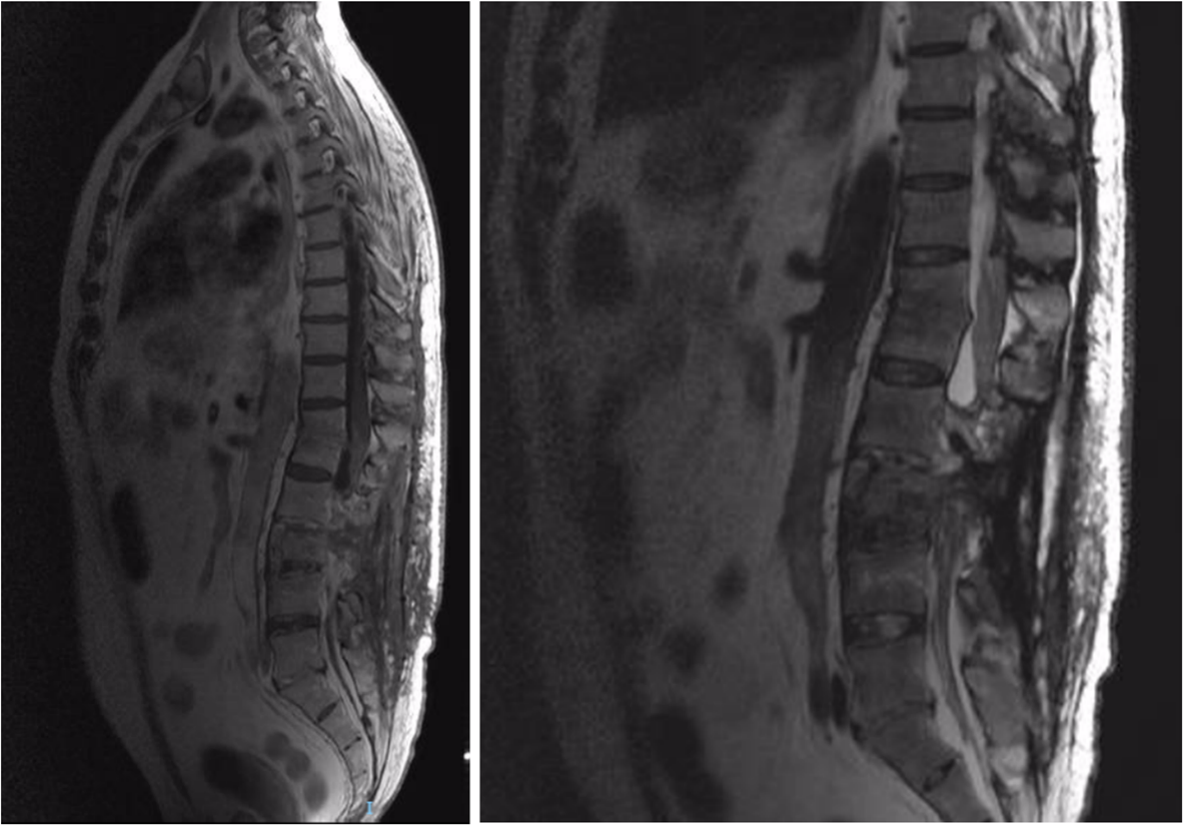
Vertebral and paravertebral infectious foci detected at vertebral magnetic resonance imaging after neurosurgical intervention for final removal of indwelling fixators
